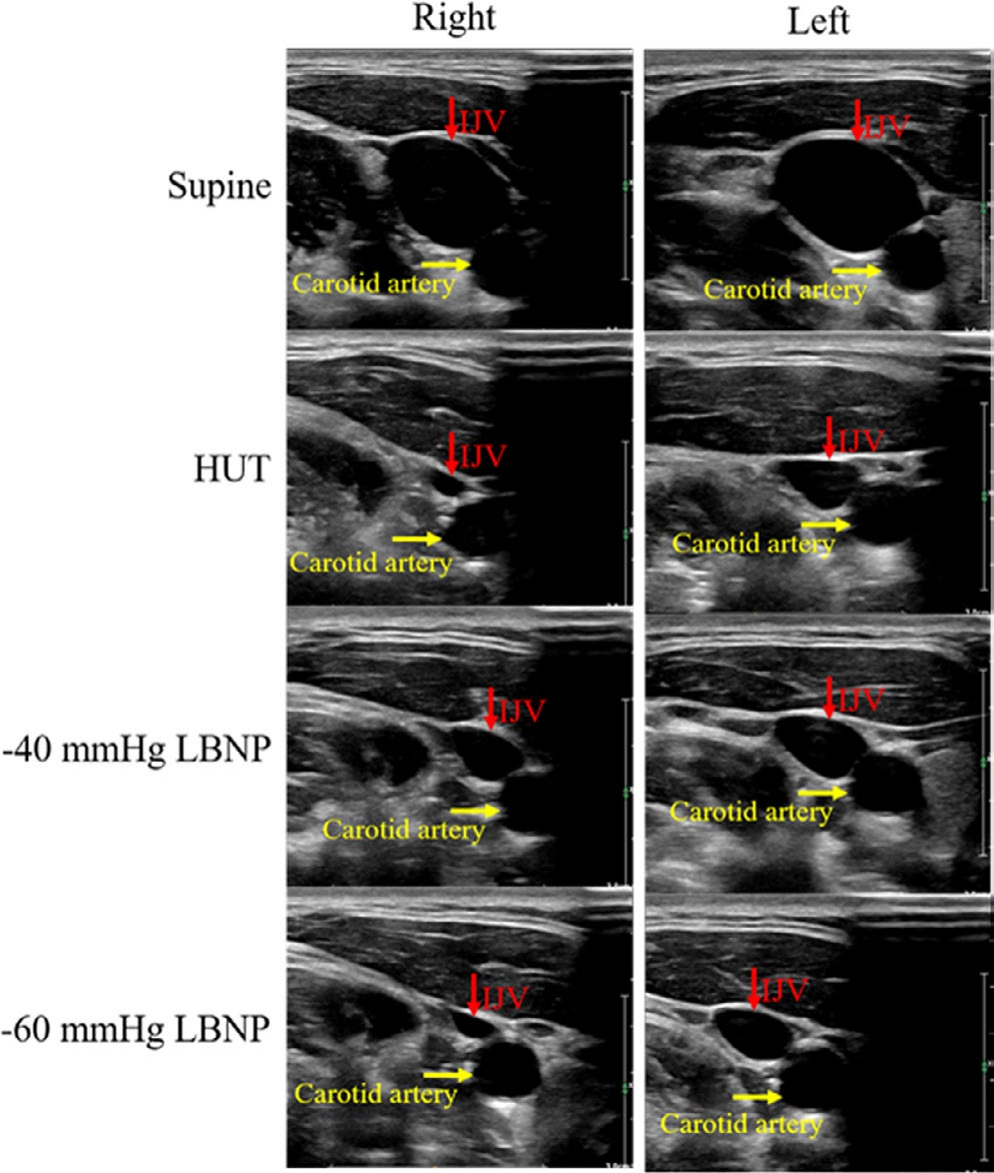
Internal jugular vein (IJV) imaging by B‐mode ultrasonography. Right and left side IJV in the supine position (top panel), 60° head‐up tilt (HUT, second top panel), −40 mmHg lower body negative pressure (LBNP, second bottom panel), and −60 mmHg LBNP (bottom panel)
